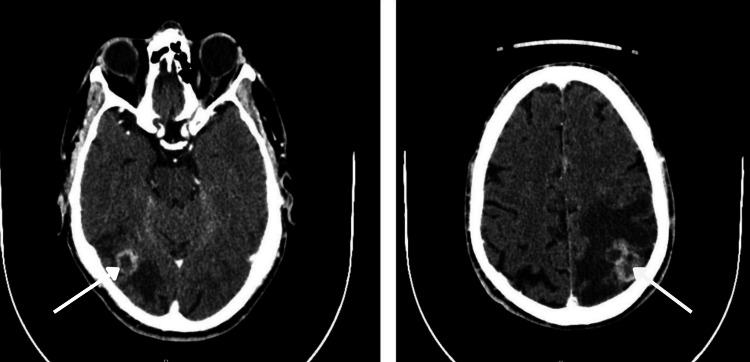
Cerebral CT scan images showing right posterior temporal (maximum diameter 17 mm) and left parietal (maximum diameter 29 mm) expansive lesions.
